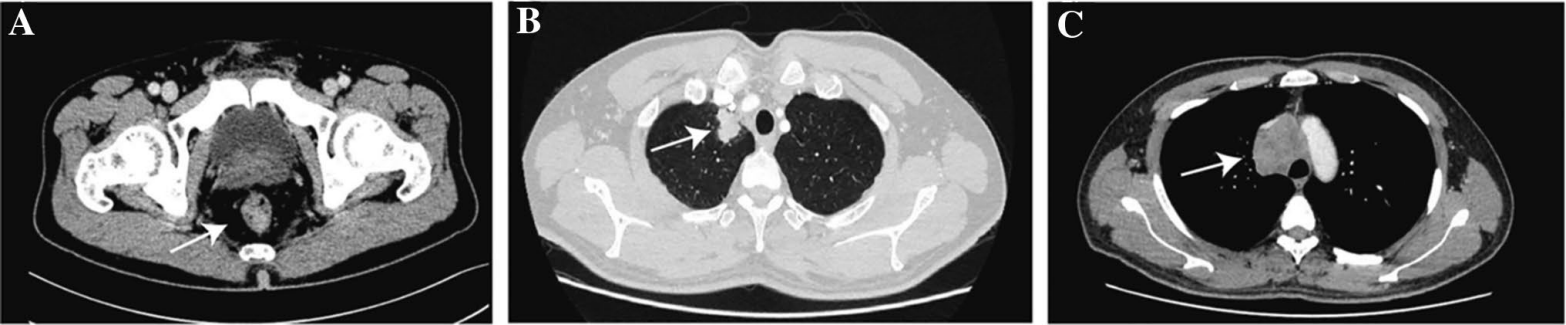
Representative CT-scan images of Case 2. A Occupation of the rectum, suggestive of rectal cancer, with invasion into the surrounding fat spaces, and no evidence of lymph-node or distant metastases observed. B, C The presence of an upper lobe right lung occupation along with enlarged mediastinal lymph nodes is indicative of malignancy and strongly suggests disease recurrence
